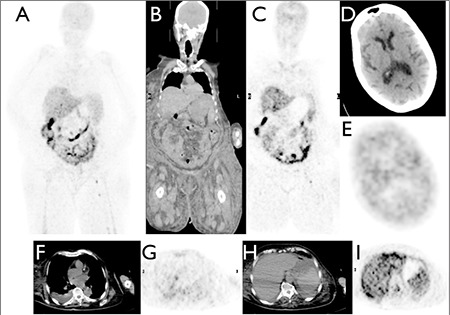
FDG PET-CT scan of the patient. A: MIP PET, B and C: coronal CT and PET, D and E: axial CT and PET at the level of the brain, F and G: axial CT and PET at the level of the heart, H and I: axial CT and PET at the level of the liver
